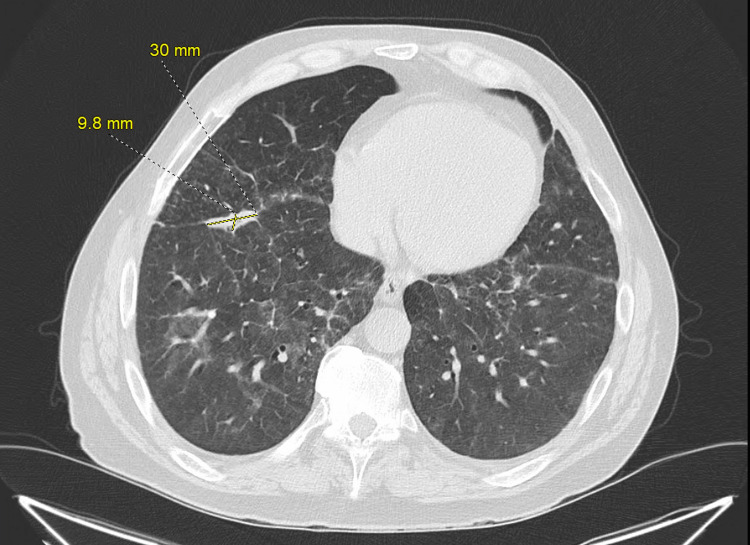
CT scan at eight months Lung nodule measuring 30 mm x 9.8 mm
